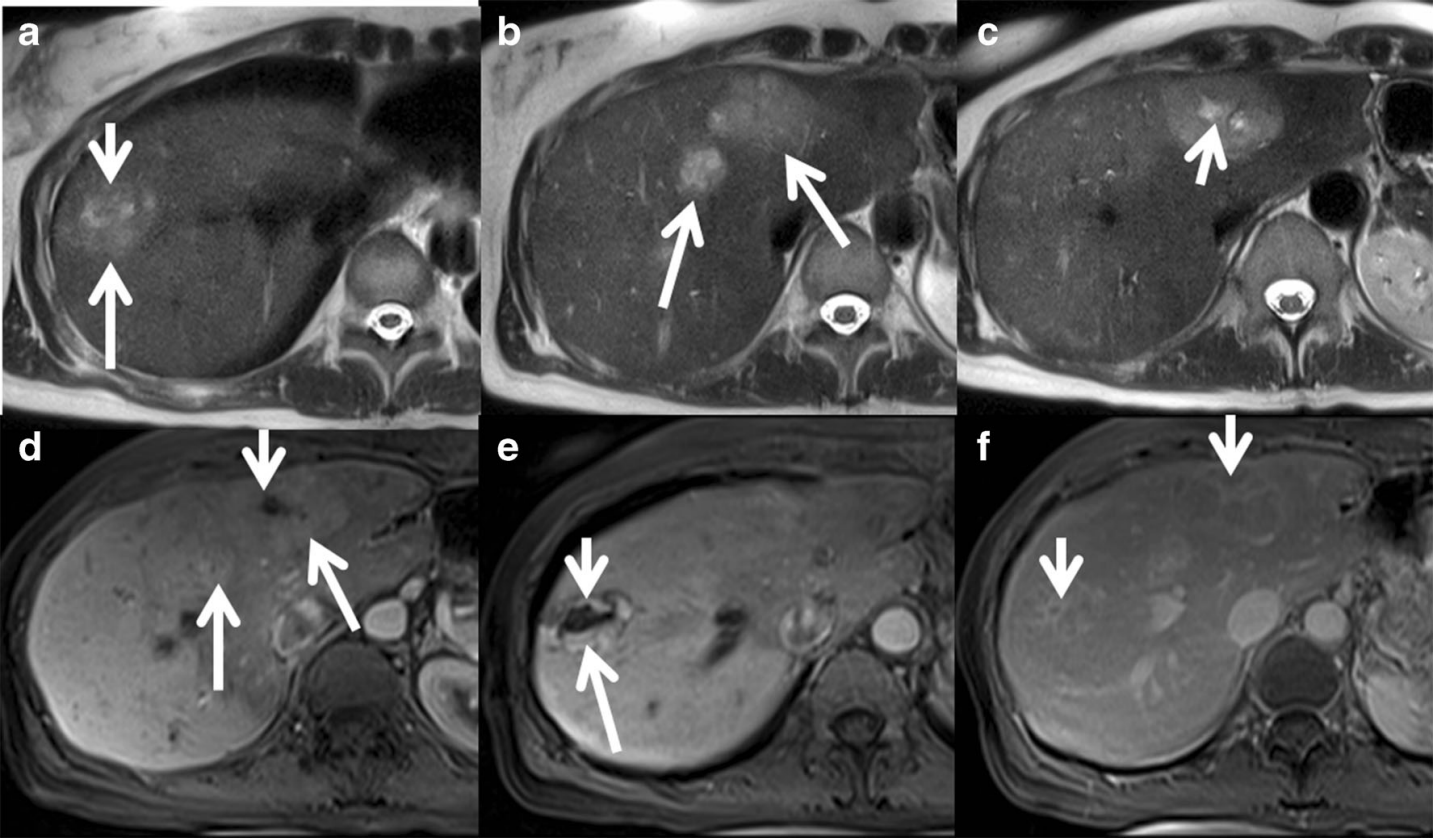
Hepatic adenomatosis in a 45-year-old male. (a), (b) and (c) Axial T2W MRI at multiple levels shows at least three mildly T2 hyperintense focal lesions (*long arrows*) in the right and left lobes of the liver with vague central T2 hyperintense areas (*short arrows*). (d) and (e) Axial post-contrast MRI in the arterial phase shows moderate enhancement of the lesions (*long arrows*) with central non-enhancing areas (*short arrows*). (f) Axial post-contrast MRI in the delayed phase shows no persistent enhancement with mild delayed enhancement of central areas (*short arrows*). Ultrasound-guided biopsy showed β-catenin-mutated hepatocellular adenoma with no dysplasia. The patient is planned for liver transplantation
